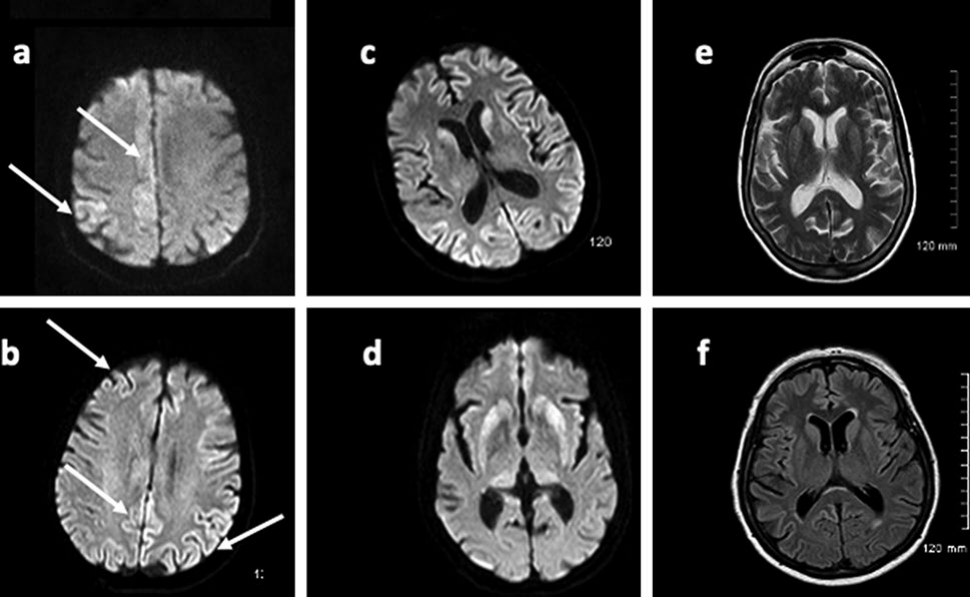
Sample images of premortem MRI brain sequences demonstrate findings suggestive of sCJD. a, b Diffusion-weighted sequences demonstrating cortical restricted diffusion (“cortical ribboning”) of bilateral parietal, cingulate and right frontal cortices; c, d diffusion-weighted sequences demonstrating restricted diffusion in bilateral caudate (c, d) and putamen (d), c also demonstrates cortical ribboning, d also demonstrates bilateral posterior thalamic diffusion restriction—an atypical finding in sCJD; e T2 and f FLAIR sequences demonstrating bilateral caudate and putamenal hyperintensity
